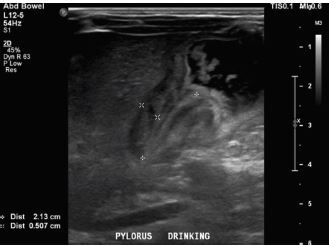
Ultrasound of pylorus with child drinking demonstrating hypertrophy (length 2.13cm and width 0.5cm) with minimum passage of fluids through the pylorus.
